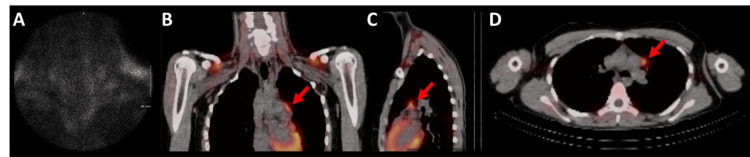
Parathyroid and thyroid scans Representative nuclear medicine studies indicating a lack of avidity in the peri-thyroid region (panel A) with a concomitant increase in metabolic activity around the prevascular mediastinal area (red arrows, panels B-D) overlayed on non-contrast CT imaging in coronal (panel B), sagittal (panel C), and axial (panel D) planes. Following intravenous (IV) administration of technetium-99m sestamibi, the thyroid (panel A) and mediastinum (panels B-D) were evaluated for increased uptake. The thyroid, demarcated by black (low activity) to white (high activity) shading (panel A), showed uptake within normal ranges. However, the mediastinum, demarcated by red (low activity) to yellow (high activity) shading, showed an unusual focus of increased uptake in the prevascular mediastinal area (red arrows, panels B-D).
